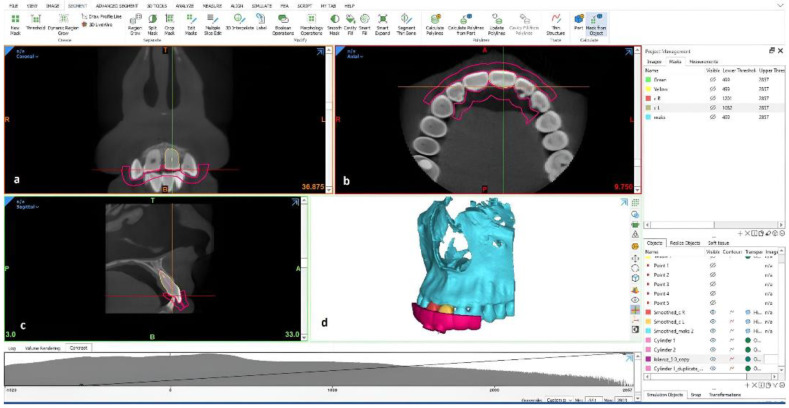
CBCT images showing 3D guide design using specific 3−matic modules and inbuilt tools. (a) Coronal plane. (b) Axial plane. (c) Sagittal plane. (d) 3D reconstruction of the maxilla and 3D guide.
